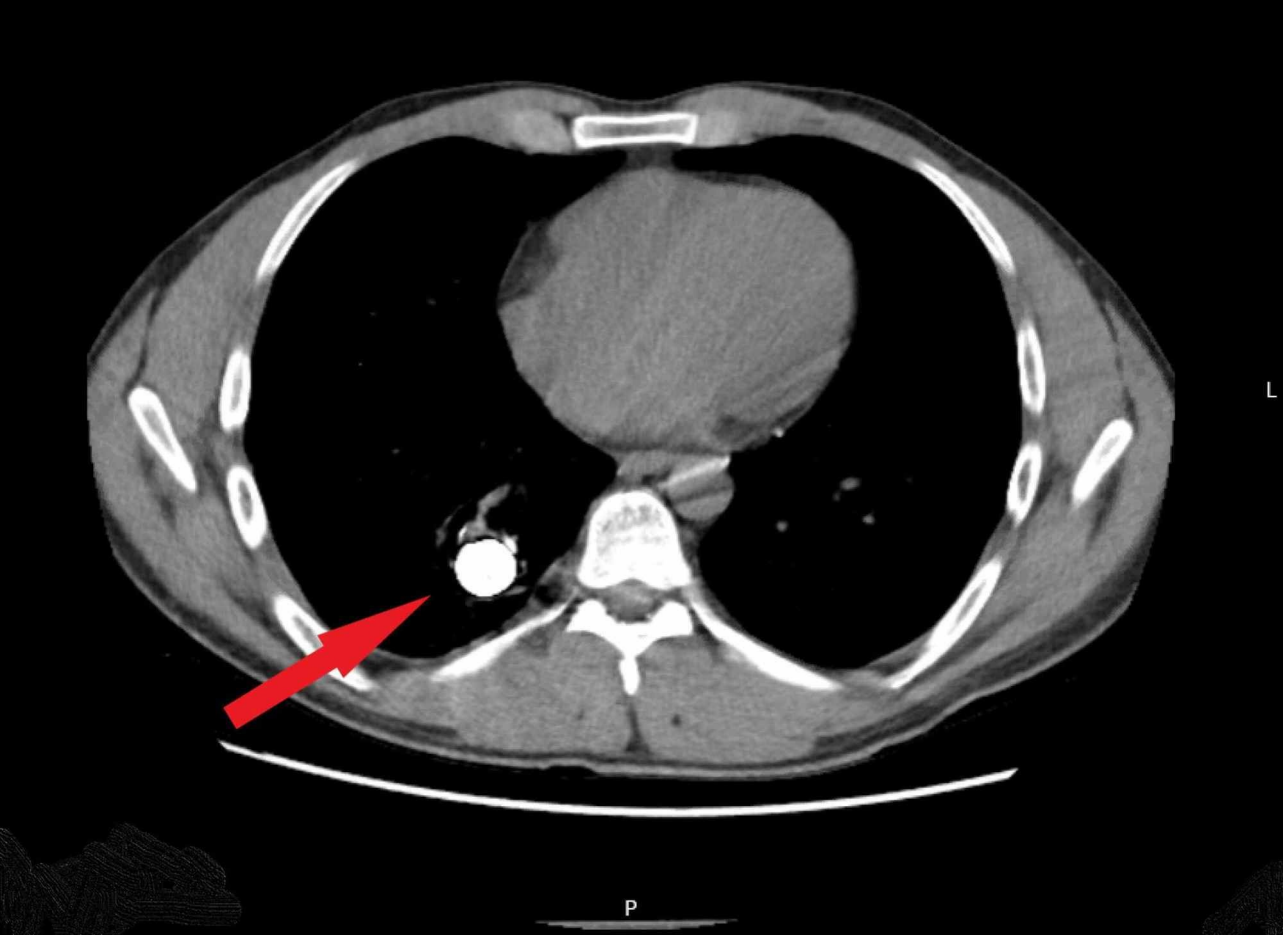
CT of the chest showing residual coil within the right lower lobe pulmonary artery aneurysm.
